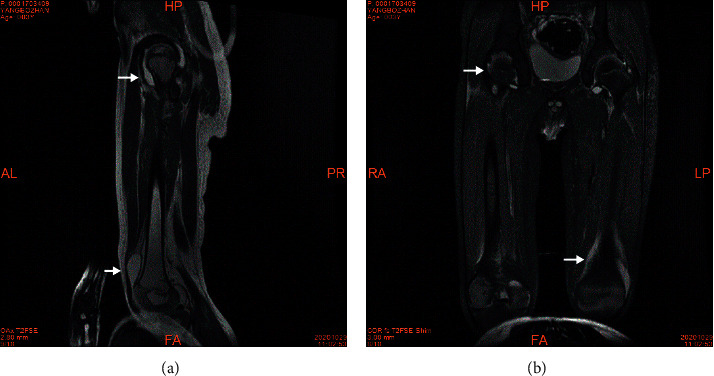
T2-weighted imaging in the acute phase of Kawasaki disease. There was a long T2 signal in the bilateral hips, knees, and bursae suprapatellaris (a), (b), which indicated arthritis with effusion, while there was an inflammatory edema signal in thigh skeletal muscle.
